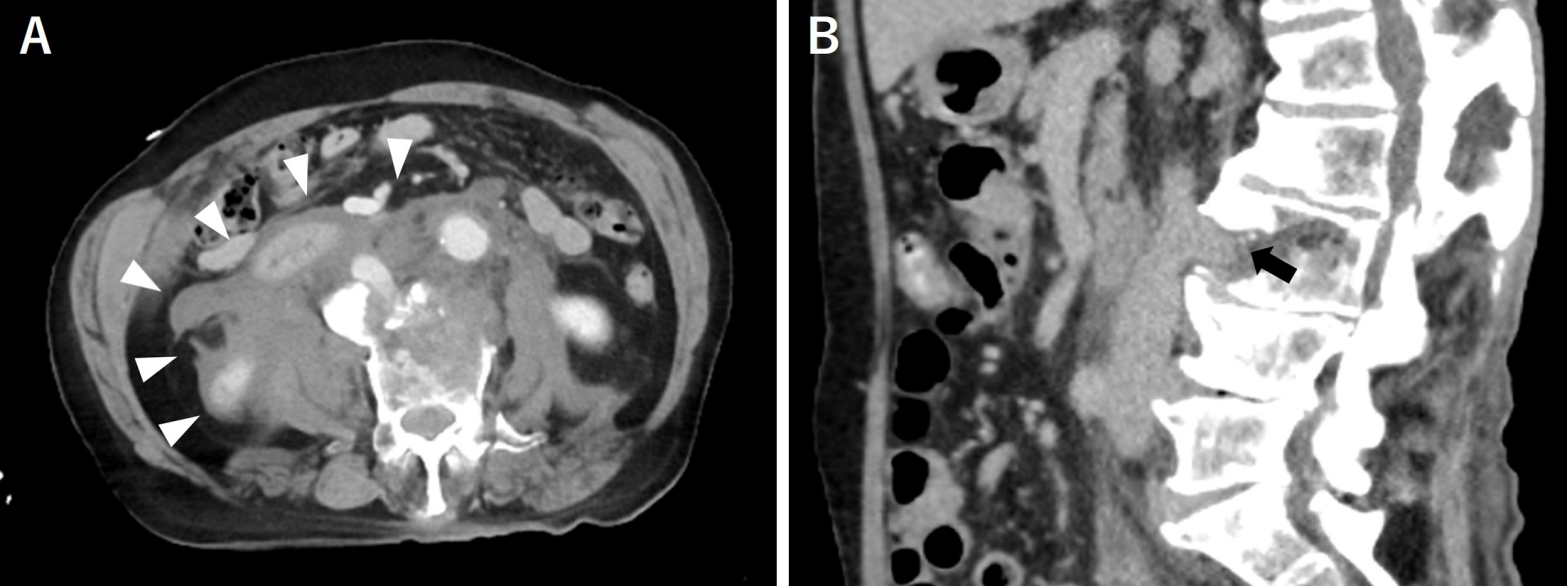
An axial slice (A) of the contrast-enhanced computed tomography revealed a large amount of hematoma in the abdominal cavity, whereas a sagittal slice (B) showed that the inferior vena cava was damaged and in contact with the fracture site.
